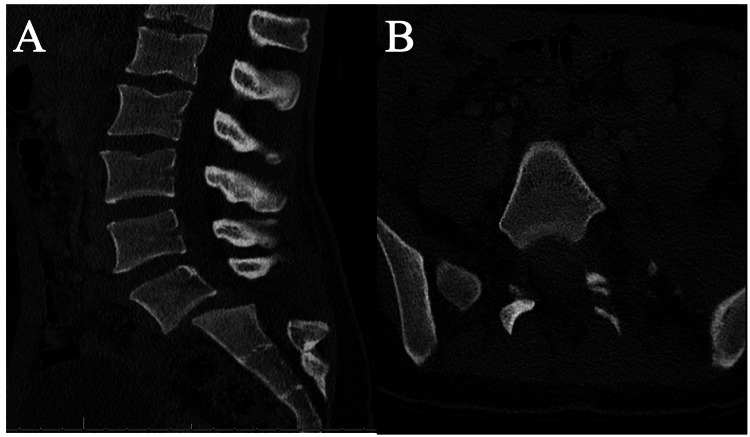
Pre-operative CT of the lumbar spine (A) Sagittal view of lumbar CT scan revealing grade 2 L5 on S1 traumatic anterior spondylolisthesis and (B) axial view of lumbar CT scan showing the S1 vertebral body and the bilateral L5 inferior articular processes in an abnormal ventral position compared to the S1 superior articular processes due to traumatic dislocation.
